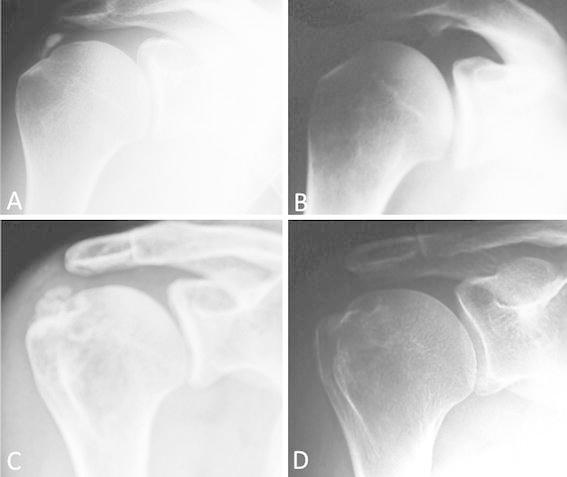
Radiographic evaluation of case 1: a preoperative X-ray (November 2005) of the right shoulder showing a subacromial (S/A) calcification of 1.2 cm; b postoperative radiographs confirmed the complete excision of the calcium deposit; c preoperative X-ray performed before the 2nd operation (February 2011) highlighted recurrence of calcification as a dense area more than 1 cm in size in the S/A space; d last follow-up radiographic evaluation confirmed the absence of ossifying mass
